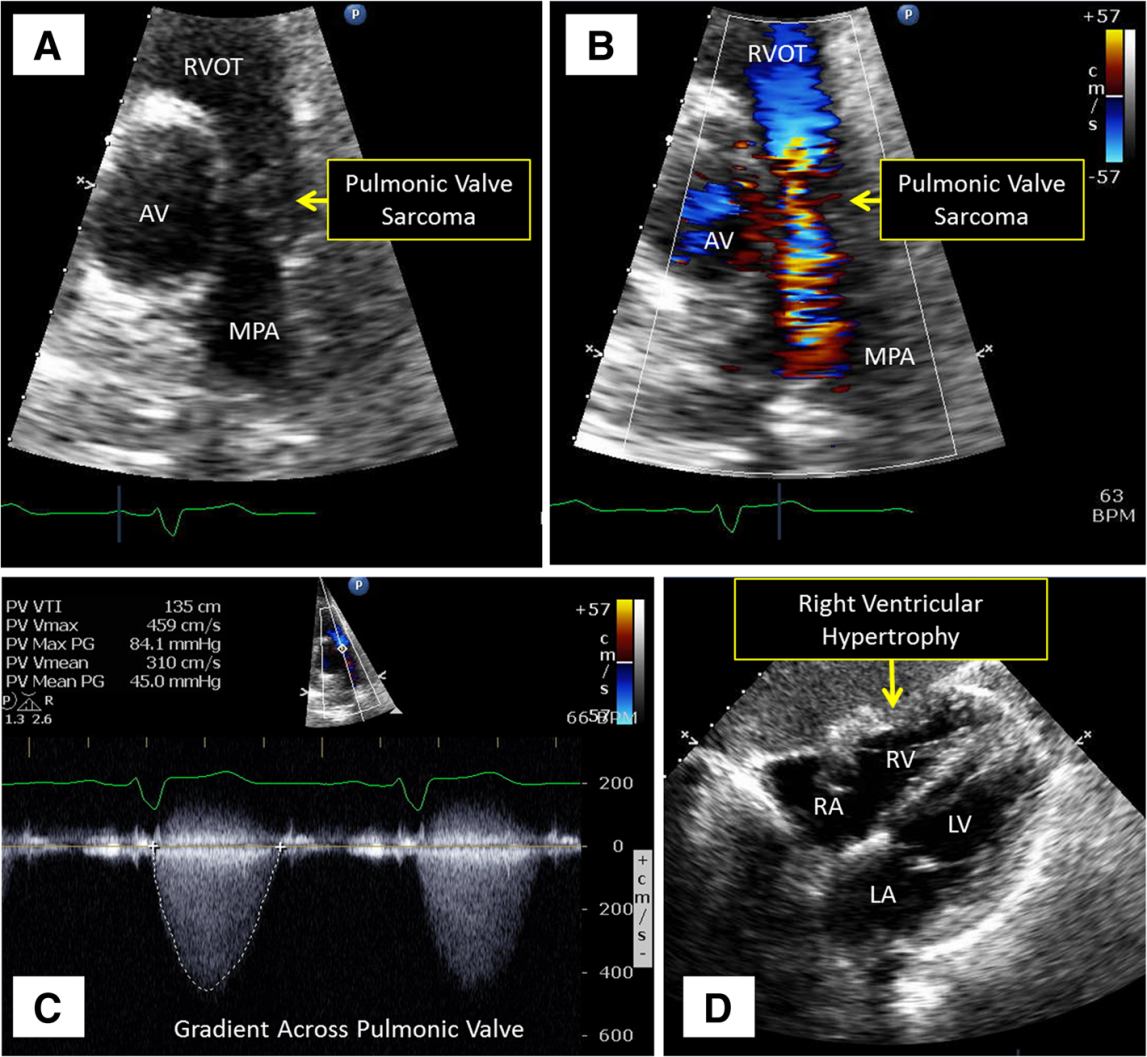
Transthoracic Echocardiogram. 2D TTE image demonstrates the sarcoma infiltrating the pulmonic valve (Panel a) leading to marked flow acceleration across the pulmonic valve on color Doppler imaging (Panel b) and spectral Doppler tracing with a peak velocity of 4.6 m/s and a peak gradient of 84 mmHg (Panel c). The presence of marked right ventricular hypertrophy (Panel d) implies chronic pressure overload of the right ventricle. Abbreviations: AV, aortic valve; LA, left atrium; LV, left ventricle; MPA, main pulmonary artery; RA, right atrium; RV, right ventricle; RVOT, right ventricular outflow tract
